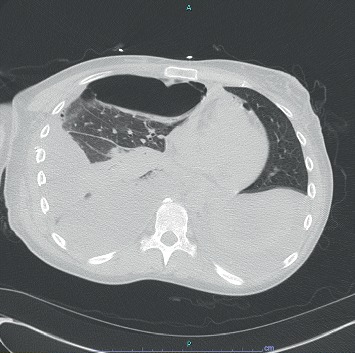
Computed tomography (CT) of the chest without contrast: moderate-large loculated right pneumothorax anteriorly with mediastinal shift to the left suggesting tension component and small volume pneumomediastinum.
